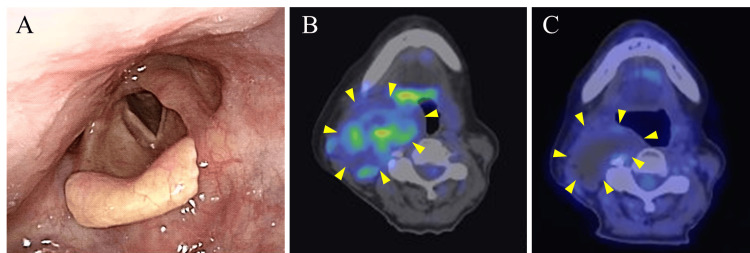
(A) Laryngeal endoscopic image three years after completion of radiotherapy. Protrusion of the right side of the oropharynx was reduced. (B) FDG-PET/CT scan before treatment. (C) FDG-PET/CT scan three years after the end of treatment. Three years after the end of treatment, the tumor had reduced, and FDG accumulation in the tumor was no longer observed. FDG-PET/CT: fluorodeoxyglucose-positron emission tomography/computed tomography
